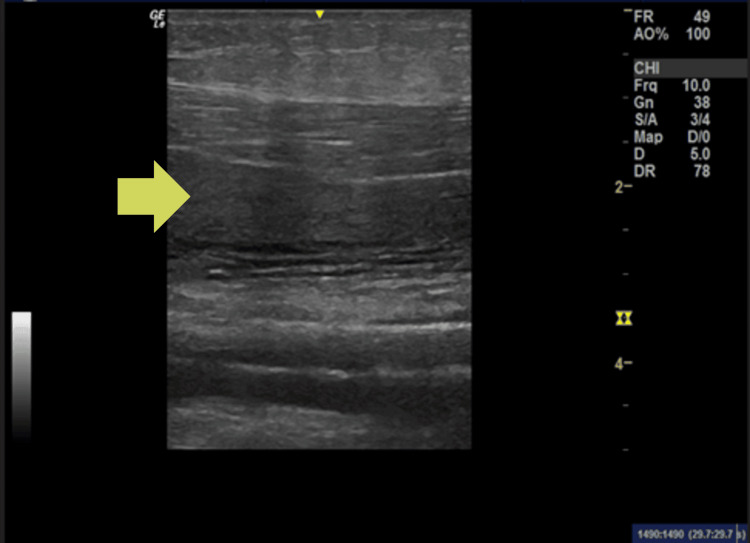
POCUS findings: the right thigh showing muscle edema with loss of striae. POCUS: point-of-care ultrasound.
